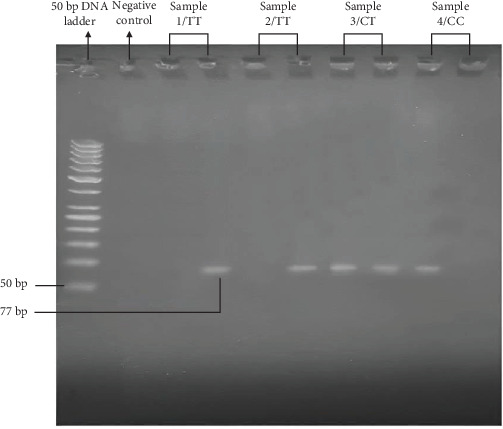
Agarose gel electrophoresis image of amplified PCR products of *FokI* gene polymorphism. Lane 1 is a 50 bp DNA ladder. Lane 2 is a negative control without any sample. Sample 1 and 2 are TT homozygous. Sample 3 is CT heterozygous. Sample 4 is CC homozygous.
